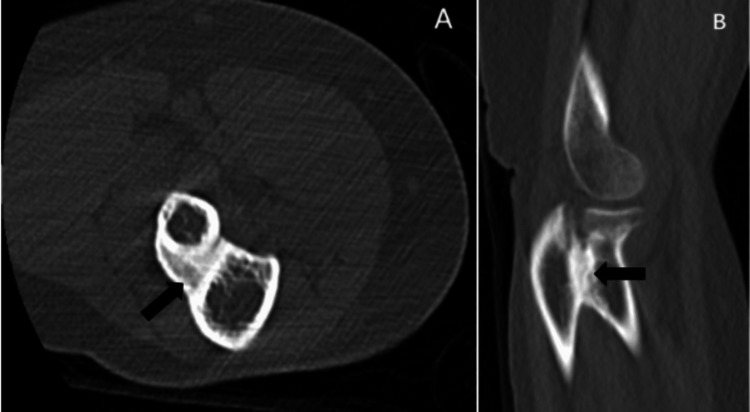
CT scan of the right elbow 18 months after the patient's injury in axial cut (A) and sagittal cut (B), with the arrow indicating the synostosis formation at the PRUJ level measuring about 2 cm and healed radial head fracture PRUJ: proximal radioulnar joint
